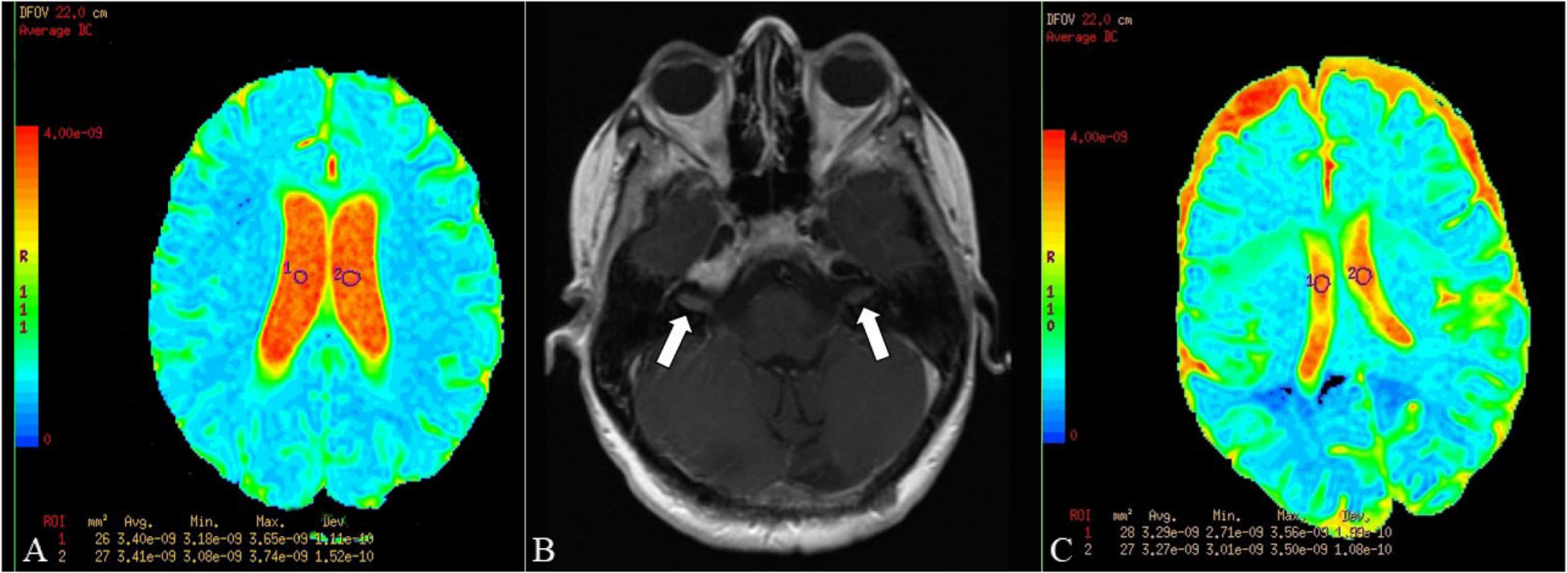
ROI placement in the mid body of the lateral ventricles in patients with LMD. a, Breast cancer with LMD. ADC values were 3.4 × 10^− 9^ m^2^/s and 3.41 × 10^− 9^ m^2^/s on the right and left side, respectively. The volume of the lateral ventricles was 60.4 cm^3^. b, Axial T1 post-image series. LMD characterized by diffuse enhancement in the bilateral internal auditory canals (arrows). c, Lymphoma with LMD. ADC values were 3.29 × 10^− 9^ m^2^/s and 3.27 × 10^− 9^ m^2^/s on the right and left side, respectively. The volume of the lateral ventricles was 13.4 cm^3^. No abnormalities were present on the T1 post-contrast or FLAIR series (not shown)
